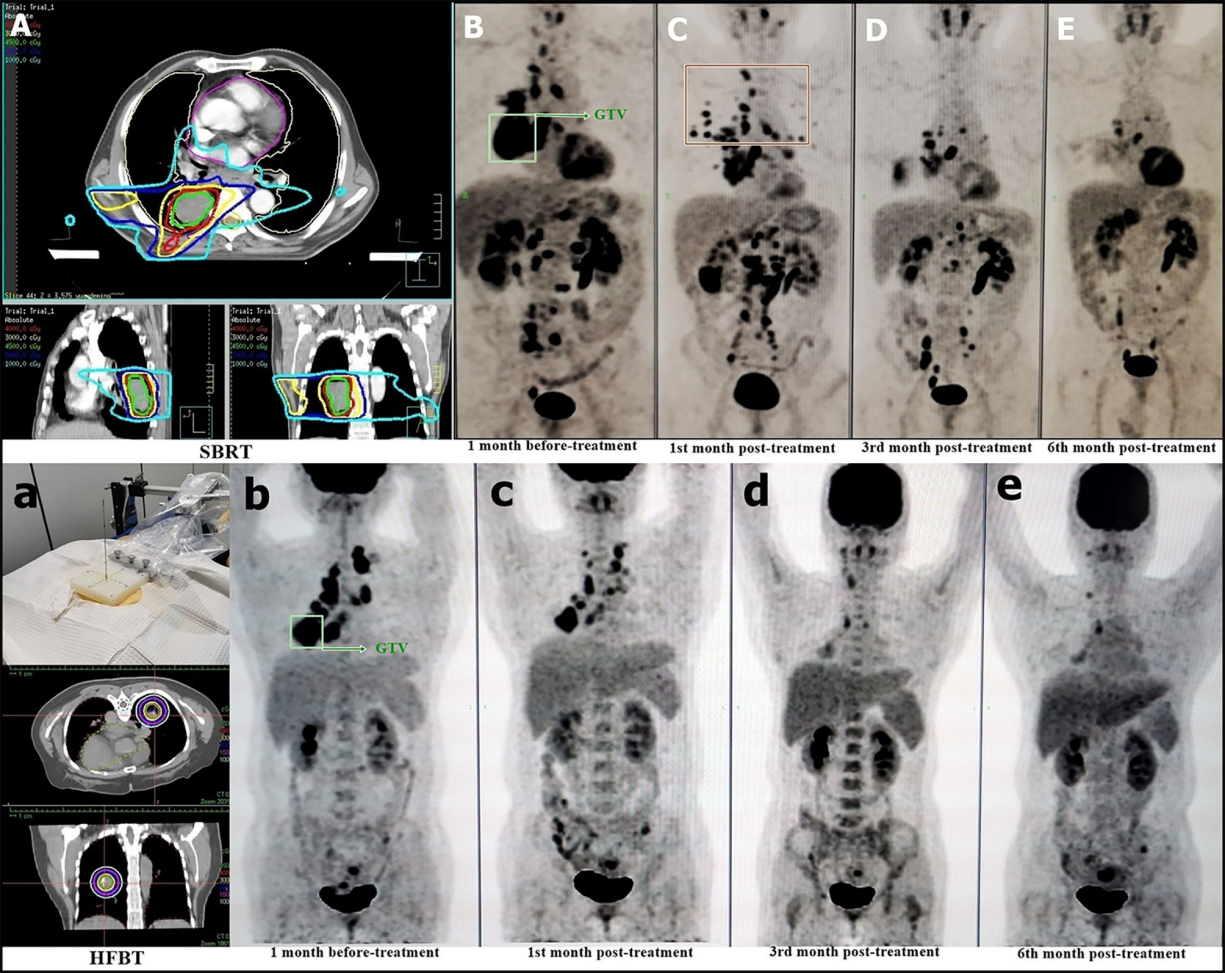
HFRT Treatment and Patient Response. The beam plan and dose distribution of SBRT on the selected lesion (A) PET–CT evaluation of pre-treatment (B) post-treatment at the first month (C), third month (D), and sixth month (E). The dose distribution of HFBT on the selected lesion (a) PET–CT evaluation of pre-treatment (b) post-treatment at the first month (c), third month (d), and sixth month (e). The green box shows the irradiated lesions for HFRT treatment (B, b).
